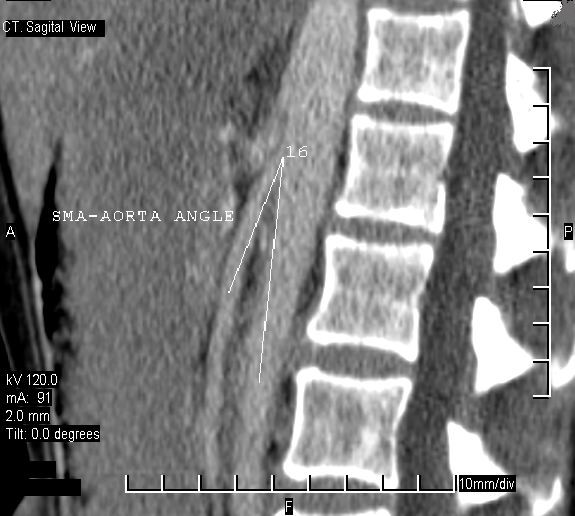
Sagittal reconstruction of CT images through the mid-abdomen showing the angle between the superior mesenteric artery and the aorta (SMA-aorta angle) is reduced to 16°.
